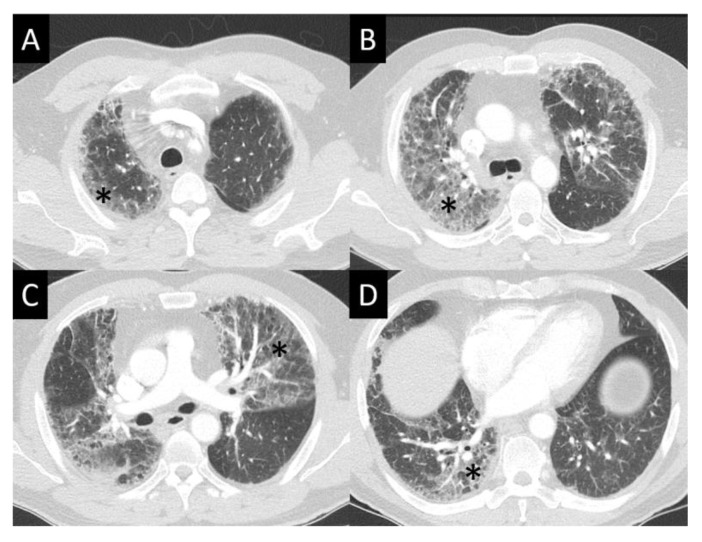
Diffuse alveolar damage (DAD). DAD is characterized by broad areas of ground glass opacity (GGO, asterisks) on CT, here seen with a geographic distribution, contrasting regions of abnormal, and normal lung (A–D).
